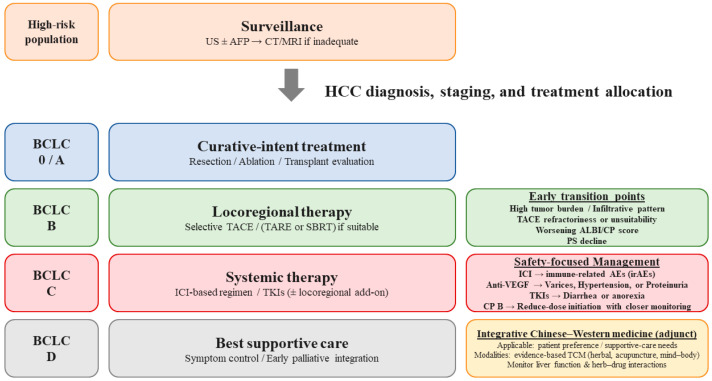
Integrated treatment algorithm for hepatocellular carcinoma. Patients at risk should undergo regular surveillance using ultrasound with or without AFP, with CT/MRI reserved for inconclusive cases. Following diagnosis, treatment strategy is primarily allocated according to BCLC stage, liver function (Child–Pugh and ALBI grade), and performance status. For BCLC 0/A, curative options including surgical resection, ablation, or transplant evaluation are recommended. For BCLC B, locoregional therapy such as selective TACE is considered first-line, while TARE or SBRT may be alternatives where available; evidence-informed integrative supportive approaches (e.g., selected standardized traditional Chinese medicine adjuncts) may be considered as optional supportive care in appropriate patients, with careful attention to hepatic safety and herb–drug interactions. For BCLC C, systemic therapy with immune checkpoint inhibitors and/or tyrosine kinase inhibitors is recommended, and locoregional therapy may be added in selected cases. For BCLC D, supportive and palliative care is indicated. Early transition from locoregional to systemic therapy should be considered in cases of high tumor burden, infiltrative pattern, TACE refractoriness, worsening liver function after local therapy, macrovascular invasion/extrahepatic spread, or decline in performance status. Treatment safety is particularly important in cirrhotic patients; immune-related adverse events (irAEs) must be monitored during ICI therapy, variceal screening is necessary when using anti-VEGF agents, and early supportive management is crucial for TKI-related toxicities. Abbreviations: US, ultrasonography; AFP, α-fetoprotein; CT, computed tomography; MRI, magnetic resonance imaging; HCC, hepatocellular carcinoma; BCLC, Barcelona Clinic Liver Cancer; TACE, transarterial chemoembolization; TARE, transarterial radioembolization; SBRT, stereotactic body radiotherapy; ICI, immune checkpoint inhibitor; TKI, tyrosine kinase inhibitor; ALBI, albumin–bilirubin; CP, Child–Pugh; irAE, immune-related adverse events; VEGF, vascular endothelial growth factor.
